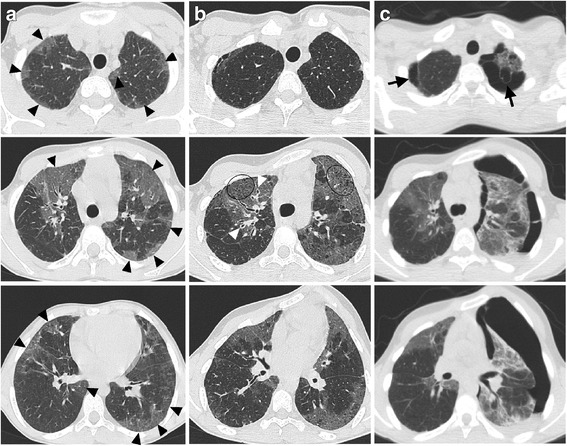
CT images of subject 3. a, b, c CT images at 8.7 years of age (column a) and follow-ups at age of 14.5 and 14.8 (column b and c). Leading pattern is GGO on both sides at initial scan (black arrowheads) and slight increase in reticular markings (encircled) and bronchial wall thickening (white arrowheads) at follow-up. Last follow-up showed distinctive pneumothorax and pleural effusion on the left and subpleural blebs (black arrows) in both lung apices
